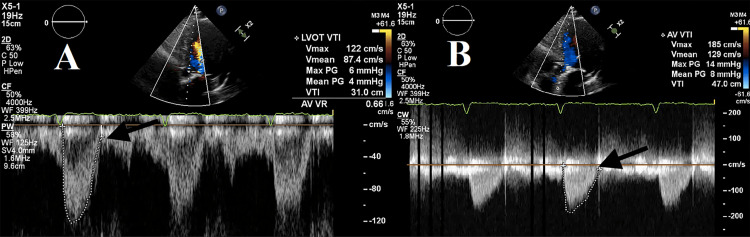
TTE spectral Doppler after TAVR of the LVOT (A) and aortic valve (B). Spectral Doppler tracings demonstrate trans-prosthetic peak velocity and dimensionless index within the normal range for valve type (1.85 m/s and 0.66, respectively). TTE, transthoracic echocardiography; TAVR, transcatheter aortic valve replacement; LVOT, left ventricular outflow tract.
